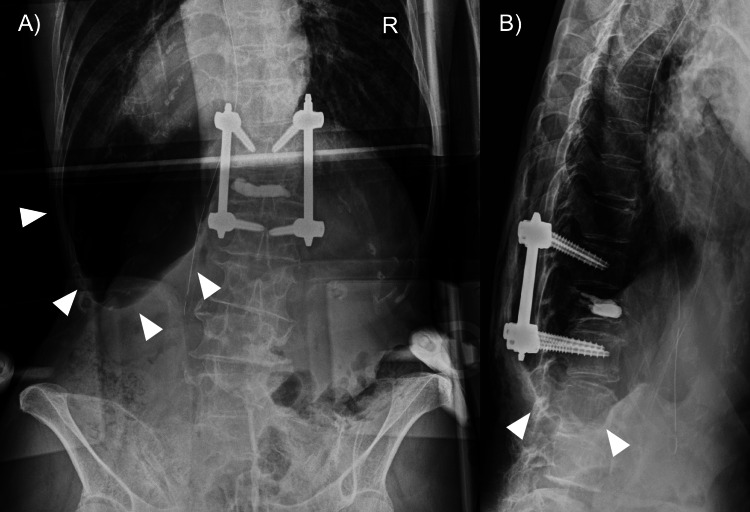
Postoperative Lumbar Spine Radiographs Showing Deep Sulcus Sign Immediate postoperative lumbar spine anteroposterior (A) and lateral (B) radiographs obtained to confirm instrumentation. Multiple arrowheads in (A) indicate a left-sided deep sulcus sign with subtle loss of vascular markings, suggesting pneumothorax.
